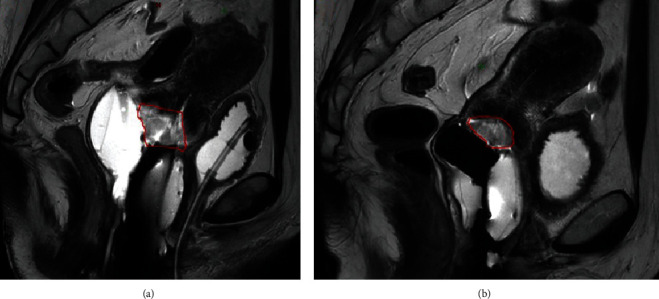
Magnetic resonance image sagittal scan with gross tumor volume (GTV) contours. (a) Fraction 1 with bulky tumor postexternal beam radiotherapy, where GTV was 20.5 cm^3^. (b) By fraction 4, there was a significant tumor response to brachytherapy with a GTV of 4.7 cm^3^.
